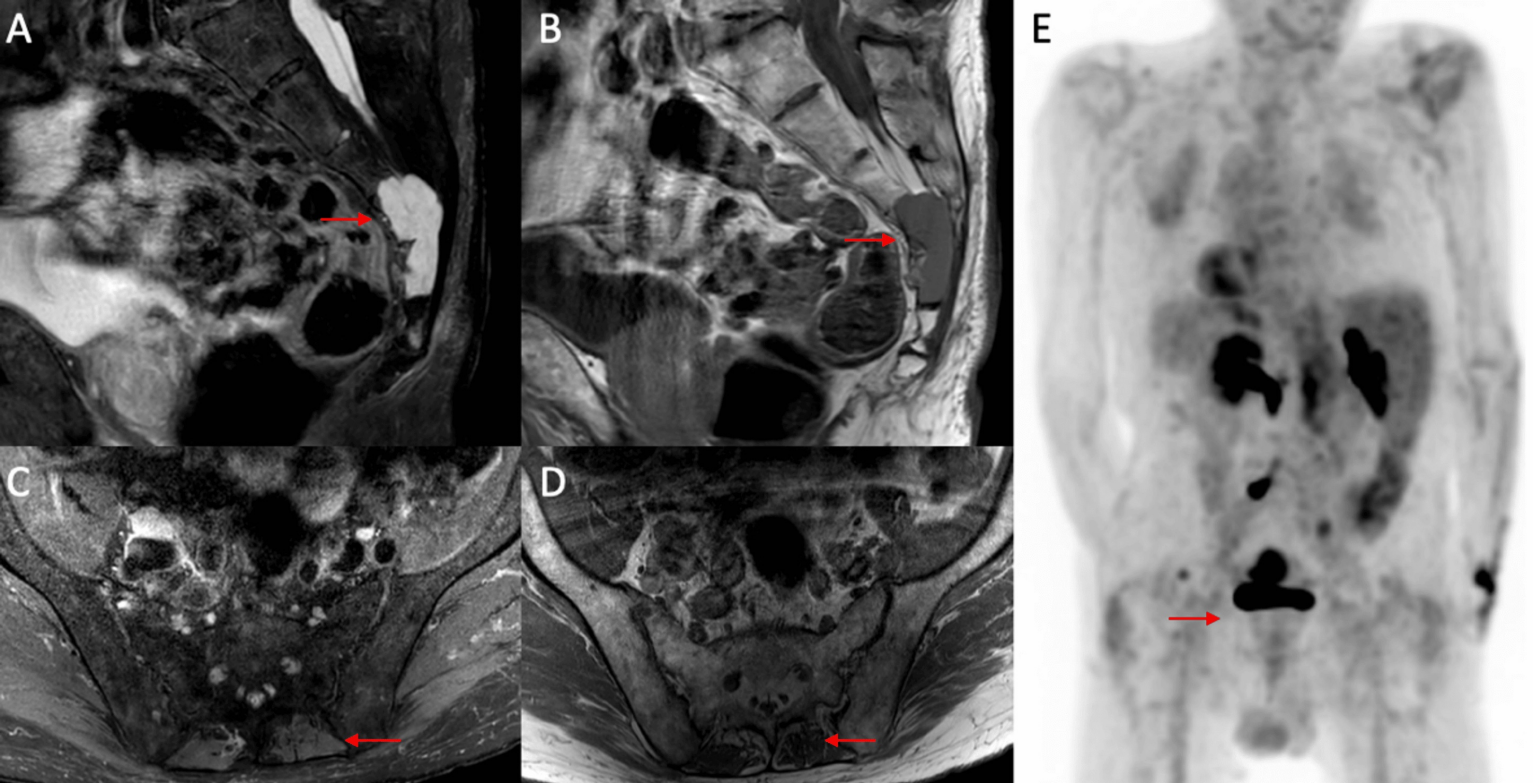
MRI of the sacral chordoma and PET scan Sagittal T2-weighted (A) and T1-weighted (B) MRI of the pelvis demonstrating a 4×2.8×1.5 cm sacral lesion from S3 to S5. Axial T2-weighted (C) and T1-weighted (D) MRI of the pelvis at S2 demonstrating no appreciable lesion in the left paraspinal musculature preoperatively. Posterior view of the preoperative PET scan demonstrating no appreciable lesion in the left paraspinal musculature (E). MRI: magnetic resonance imaging; PET: positron emission tomography
